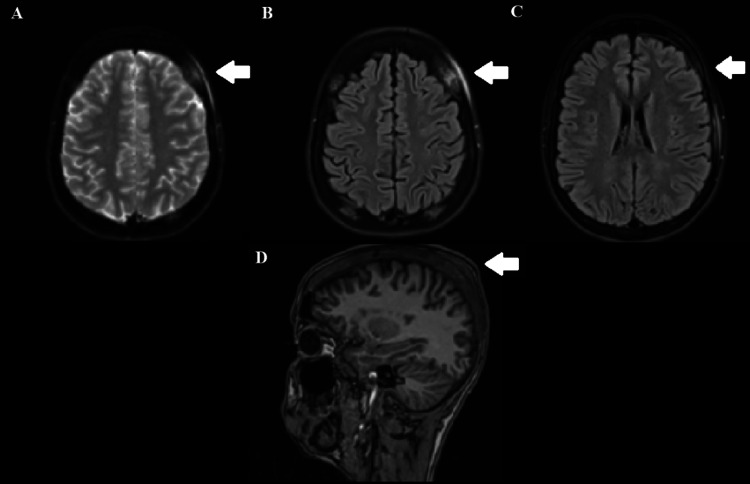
MRI sequences demonstrating calvarial and extracranial changes consistent with ASHS and possible bone infarction ASHS: acute soft head syndrome; FLAIR MRI: fluid-attenuated inversion recovery magnetic resonance imaging (A) Axial T2-weighted MRI showing diffuse expansion of the calvarial bones with patchy areas of abnormal marrow signal. (B) Axial FLAIR MRI demonstrating peripheral marrow enhancement and heterogeneous signal intensity, suggestive of early bone infarction or osteomyelitis. (C) Axial FLAIR MRI at a lower slice demonstrating small, noncontiguous subgaleal and epidural collections, more prominent at the frontoparietal region. (D) Sagittal T1-weighted MRI demonstrating marginal enhancement of the subgaleal collection and reactive changes in the overlying soft tissues. Major intracranial vessels appear unremarkable, with no evidence of territorial ischemia on MR angiography Magnetic resonance imaging (MRI) demonstrating diffuse expansion of skull calvarial bones with multiple bone marrow patches of abnormal signaling, predominantly peripheral enhancement, suggestive of potential bone infarction or osteomyelitis. Further noted a few small noncontiguous subgaleal and epidural collections, with the most significant at the frontoparietal region, revealing marginal enhancement, suggestive of tissue reaction or infective changes. Unremarkable MR angiography findings, with no evidence of ischemic insult to the major territories
